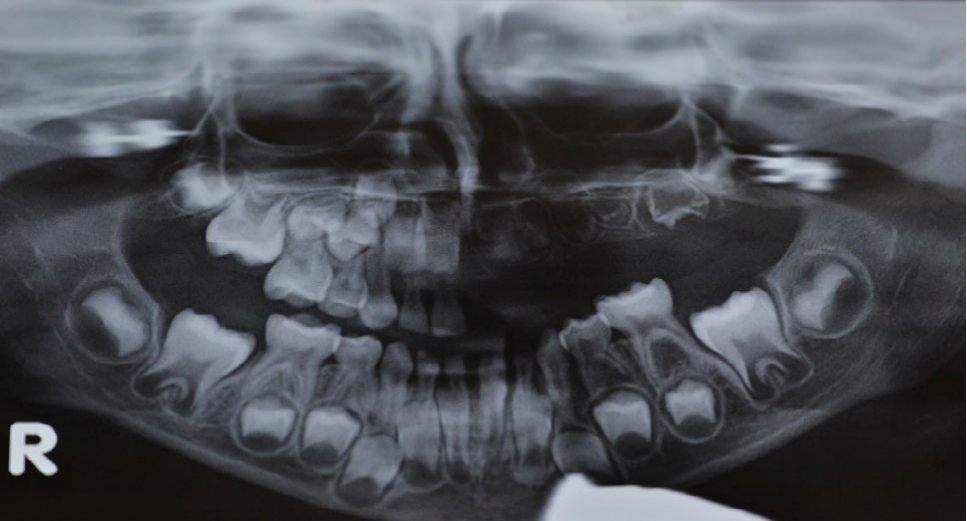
Orthopantomogram of the patient at age 6.
